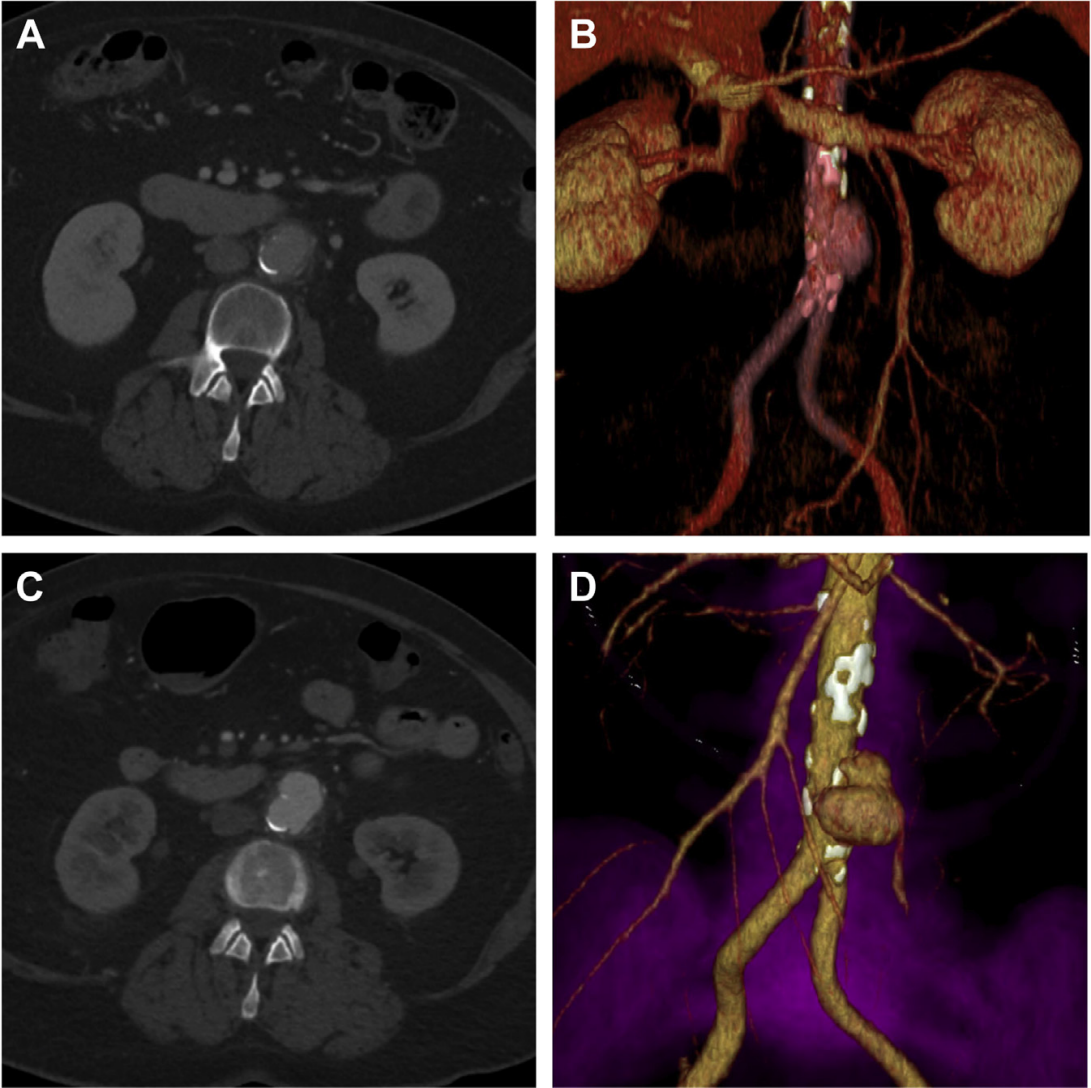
Preoperative computed tomography (CT) imaging. A, Initial CT scan with contrast demonstrating a mildly dilated aorta with a maximal diameter of 3.0 × 2.7 cm and localized inflammatory changes. B, Corresponding volume rendering image of the aortic aneurysm. C, Subsequent CT angiogram (CTA) 2 days later showing an increase in maximal measurement to 3.6 × 3.0 cm in addition to a penetrating ulcer and para-aortic stranding. D, 3D reconstruction of the CTA displaying the enlarging aneurysm.
